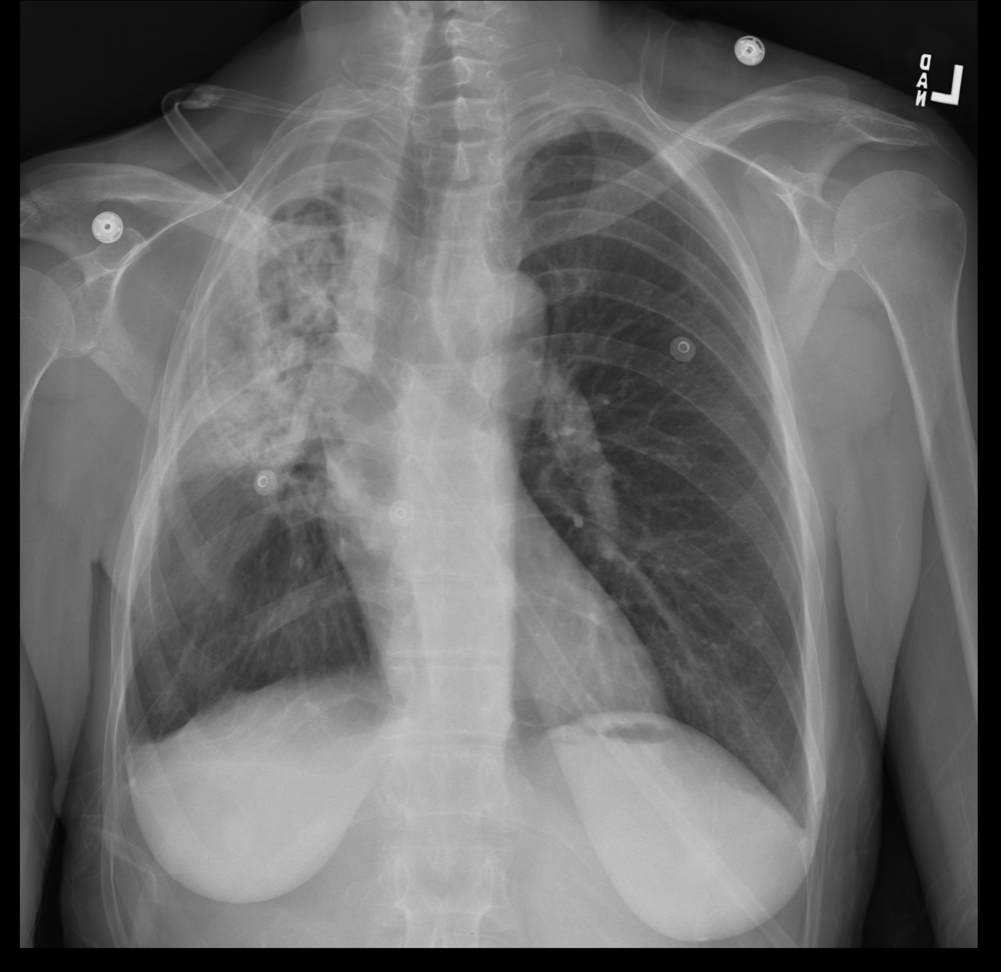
Chest radiograph obtained April 2008, approximately 2 years after pleurectomy with hyperthermic intraoperative intrapleural chemotherapy. A persistent contraction from severe fibrosis of the right upper lobe is seen.
